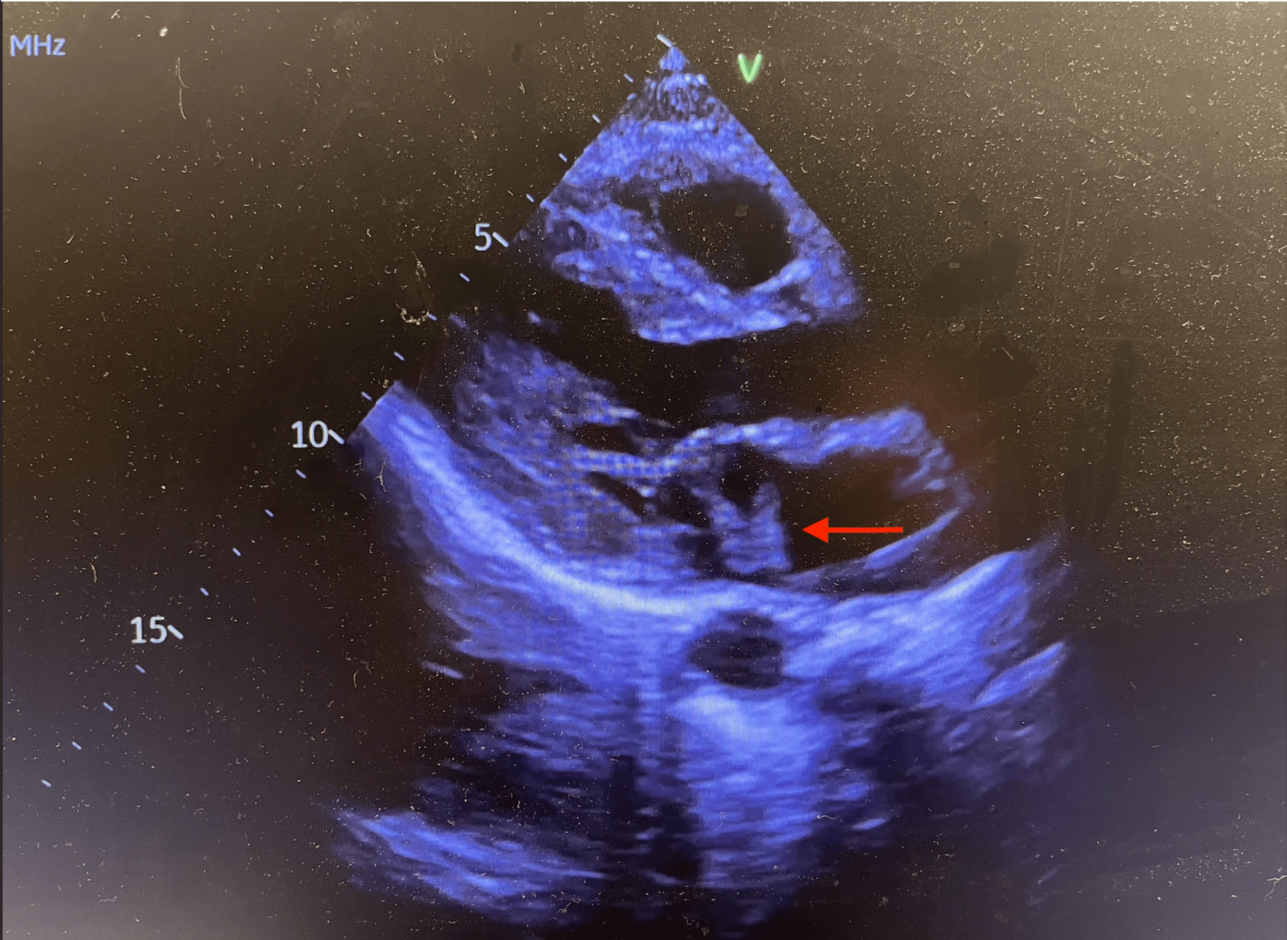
Bedside point-of-care echocardiogram showing a 4.6 cm × 1.3 cm echodensity attached to the anterior leaflet of the mitral valve, traveling freely into the left ventricle and atria, concerning for endocarditis. Significant mitral valve regurgitation is also noted, likely from the anterior leaflet vegetation.
